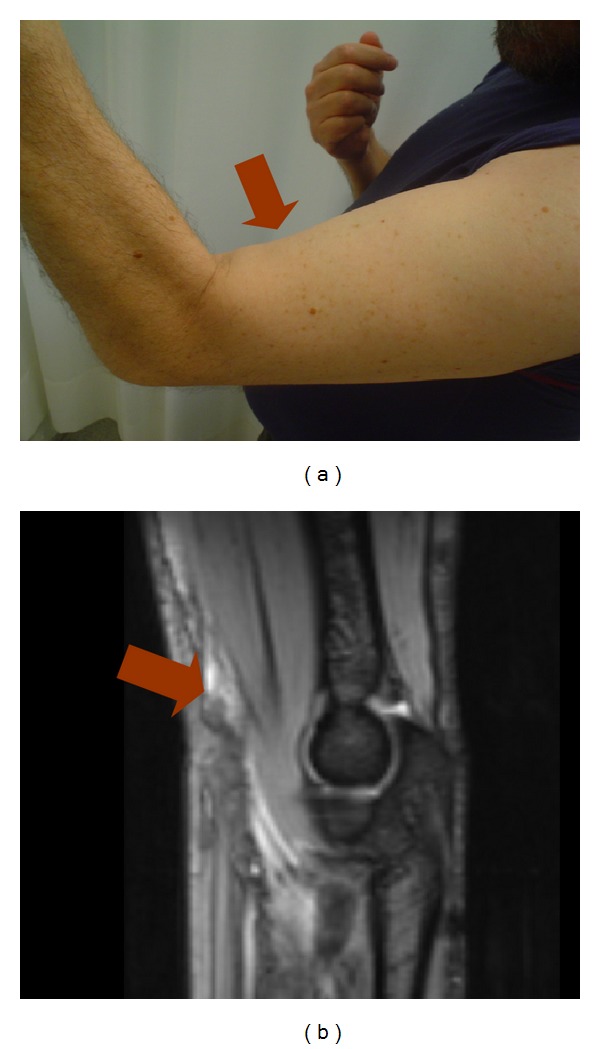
Clinical and MRI image of the myotendinous rupture of the biceps brachii muscle.
